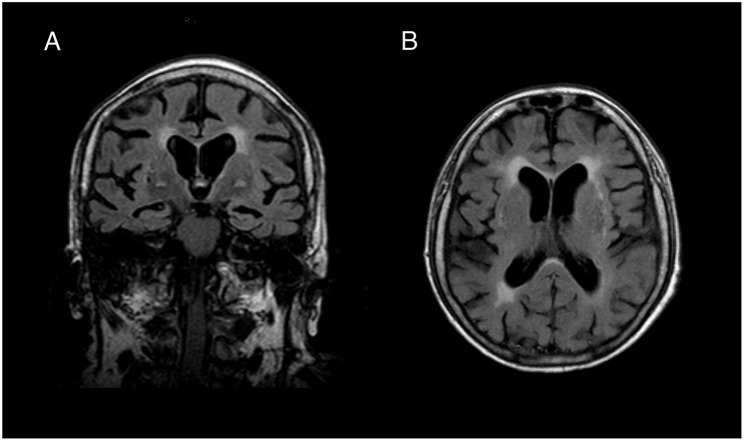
Brain MRI. (A) Coronal and (B) transversal slice of T1-weighted brain imaging revealed moderate temporal lobe atrophy (medial temporal lobe atrophy score 2) and white matter lesions (Fazekas grade 2). We did not find any indication for inflammatory processes in FLAIR, T2- and Diffusion weighted sequences.
